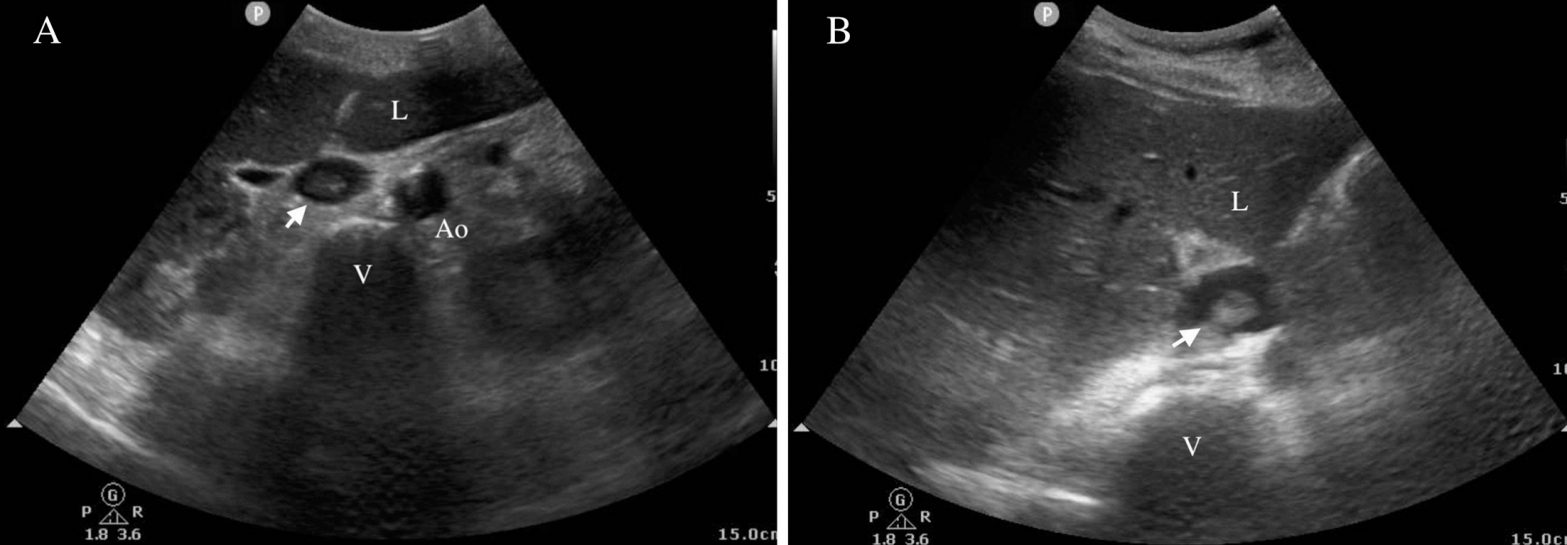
Sonographic image of the thrombi in the IVC. Thrombi in the IVC of patients 1 (A) and 2 (B). Both thrombi were hyperechoic, large, mobile, and mural (white arrows). Ao: aorta; IVC: inferior vena cava; L: liver; V: vertebral body.
